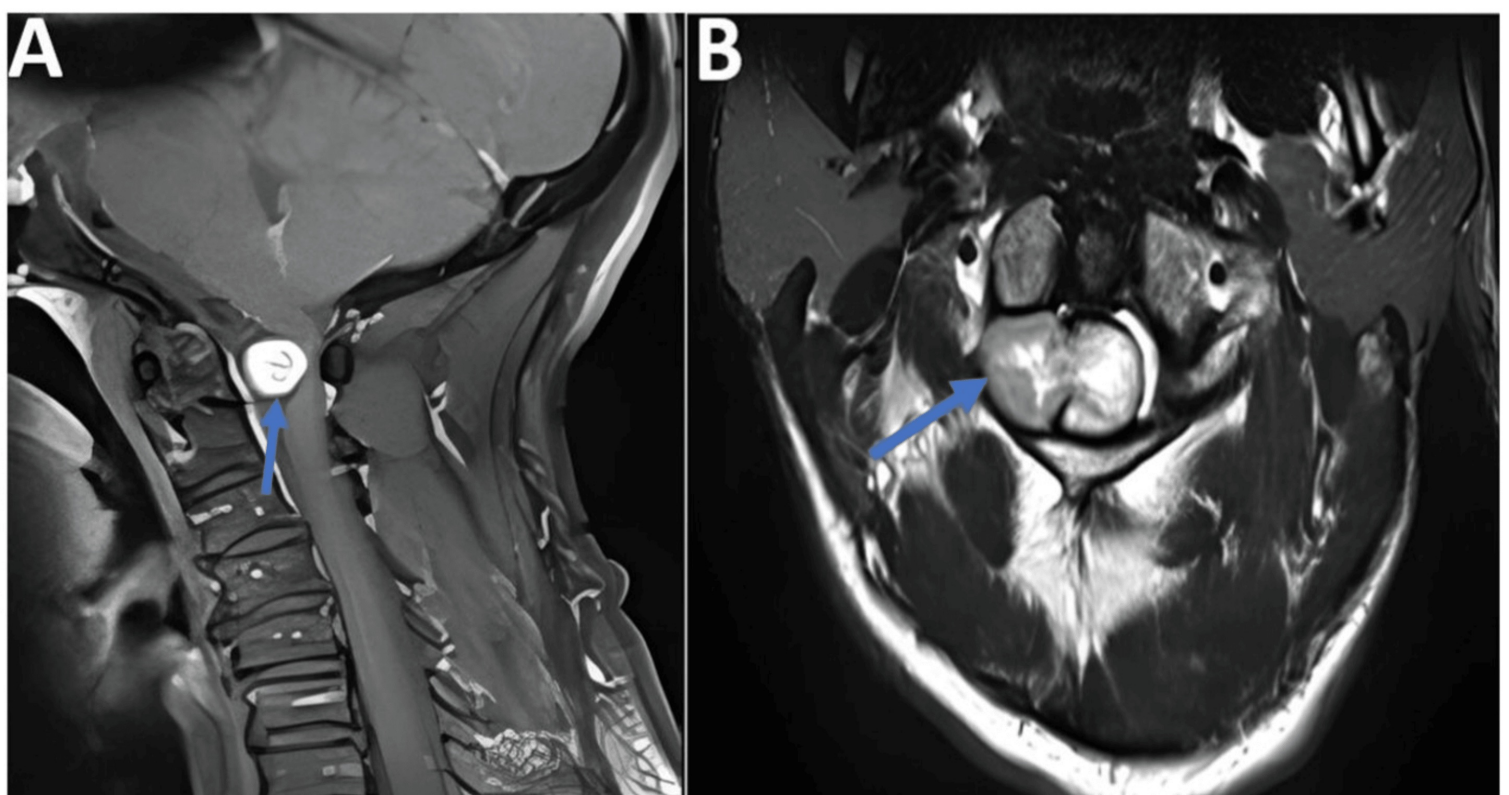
(A) Sagittal T1-weighted MRI showing an anterior intradural extramedullary mass at the level of C2 and C1; (B) Axial T1-weighted MRI showing a right anterolateral intradural extramedullary mass in contact with the C2 nerve root (blue arrow).
